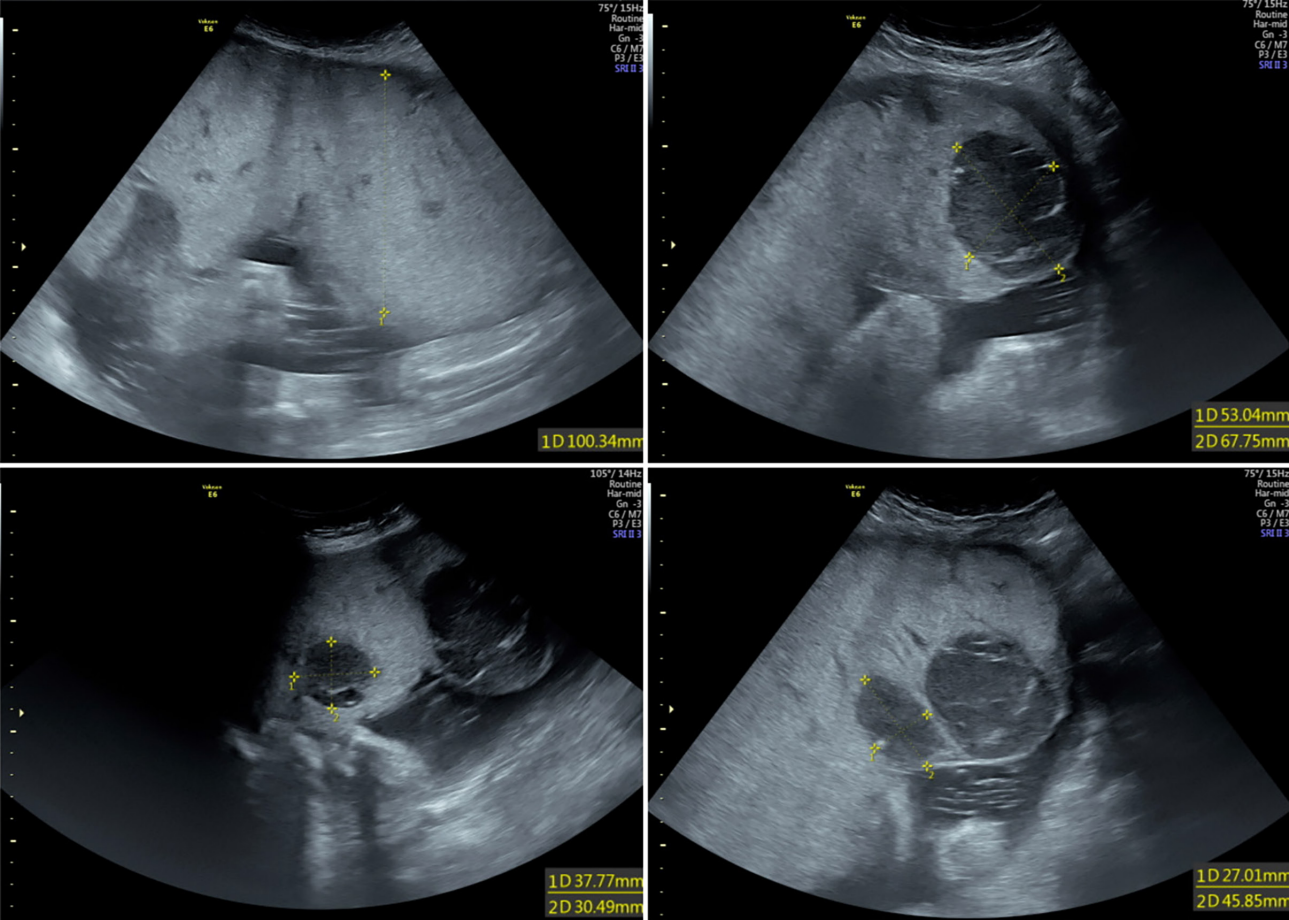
The ultrasound reveals six distinct hypoechoic lesions within the placenta.
